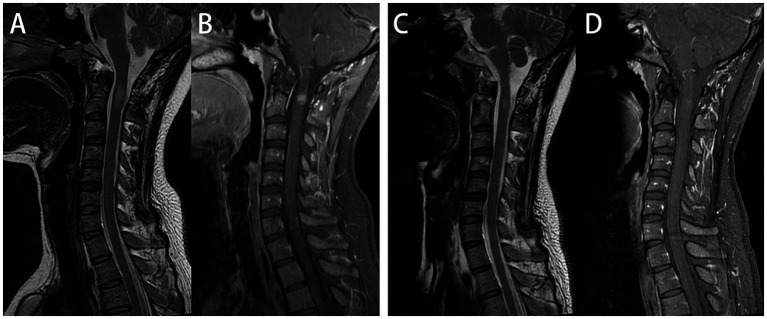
Cervical spinal cord plain and enhanced magnetic resonance imaging (MRI). On admission, cervical spine MRI demonstrated intramedullary abnormal signals at the C2 level, with nodular uniform enhancement (A,B). One month later, follow-up cervical spine MRI showed that One month later, follow-up cervical spine MRI showed that a significant reduction in both the extent of the T2WI hyperintense and enhancing lesions (C,D).
